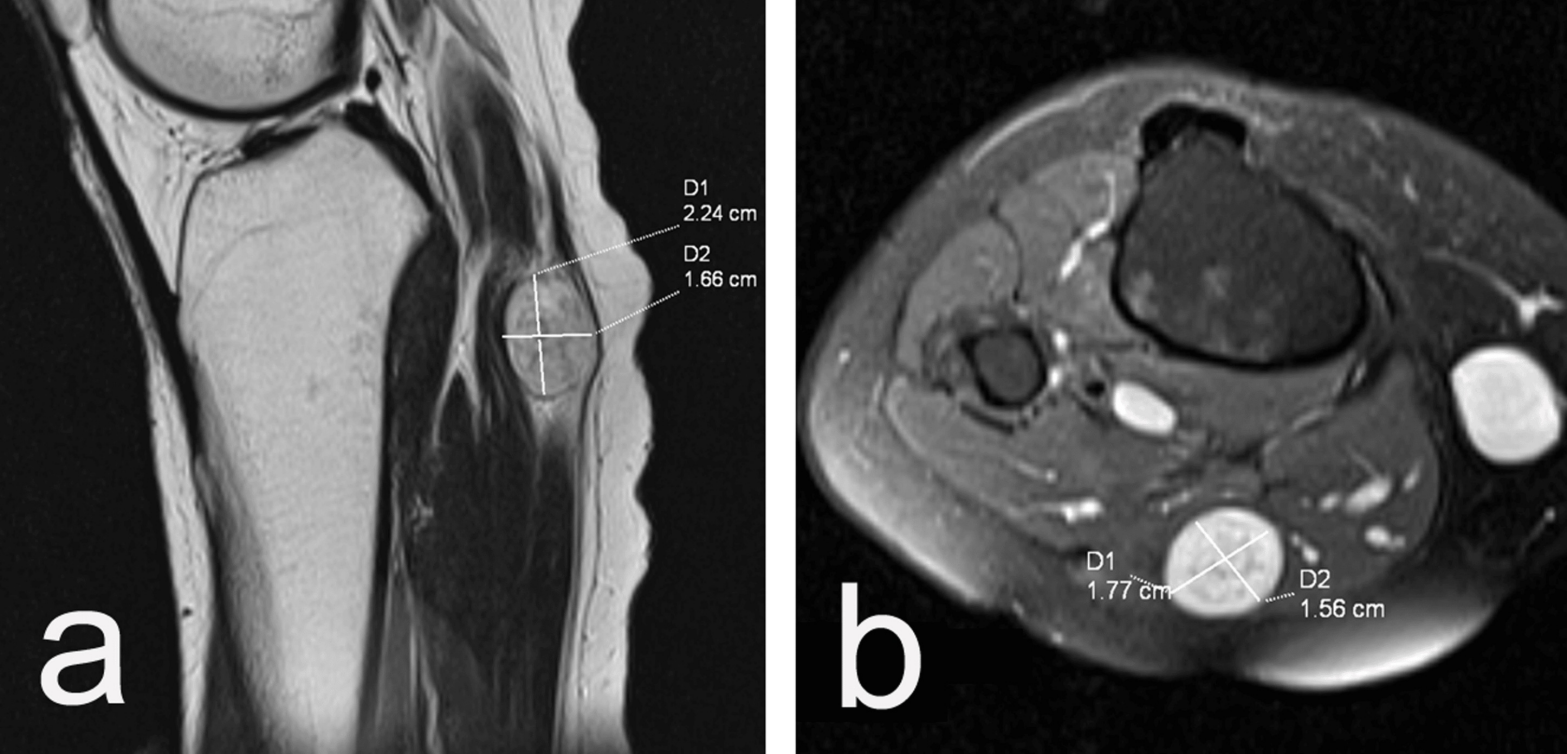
Preoperative MRI showing an oval-shaped subcutaneous mass situated in front of the gastrocnemius muscle (a, b).
